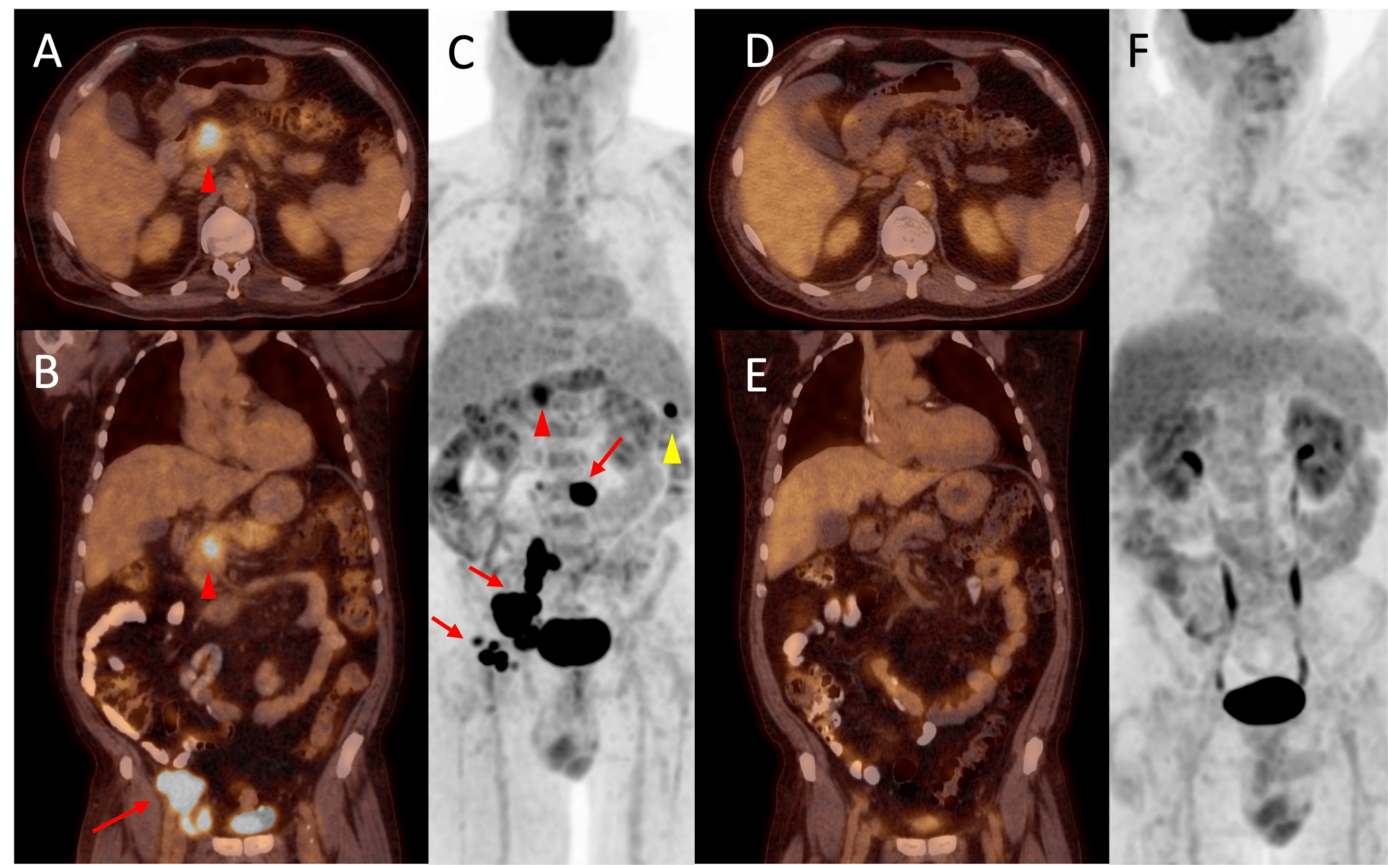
Fused PET/CT images with axial view of the chest and coronal view of the chest/abdomen/pelvis, along with coronal MIP images, before and after chemotherapy Images (A), (B), and (C) demonstrate hypermetabolic lesions in the uncinate process of the pancreas (red arrowhead), spleen (yellow arrowhead), and multiple lymph nodes, including the left para-aortic, right iliac, and right inguinal nodes (red arrows). Following three cycles of chemotherapy, images (D), (E), and (F) demonstrate resolution of the previously noted hypermetabolic activity. PET/CT: Positron emission tomography/computed tomography; MIP: Maximum intensity projection
